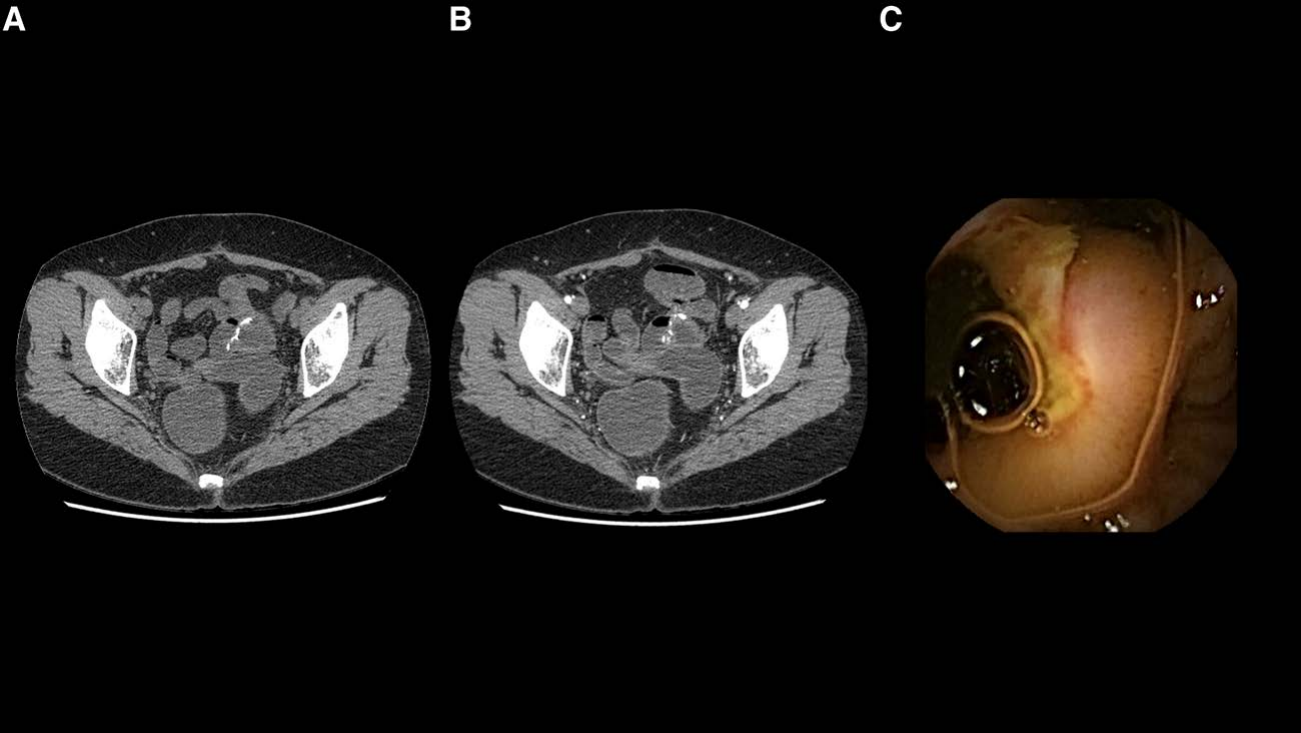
Figure 4A. Axial noncontrast enhanced CT shows patency of ileoileal anastomosis with the presence of high-density surgical materials. Figure 4B. Axial contrast enhanced CT shows no definite abnormal enhancement at anastomosis. Figure 4C. VCE showed a large clean-base ulcer at the anastomosis. CTE = computed tomography enterography, VCE = video capsule endoscopy.
